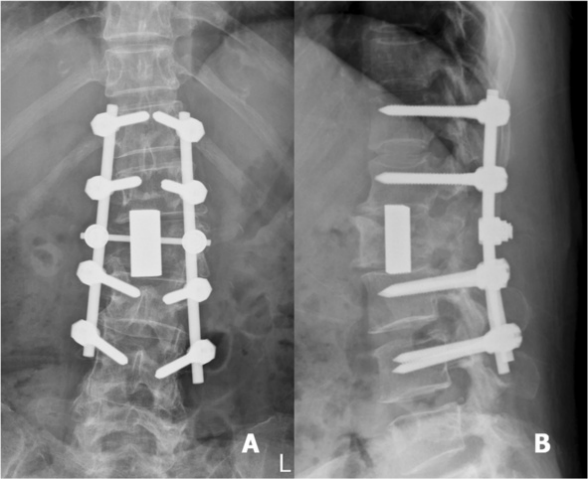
Post-operative anteroposterior (a) and lateral (b) radiographs revealed an acceptable spinal alignment that was restored with an adequate length of cervical TM mesh cage implantation and posterior pedicle screw fixation
